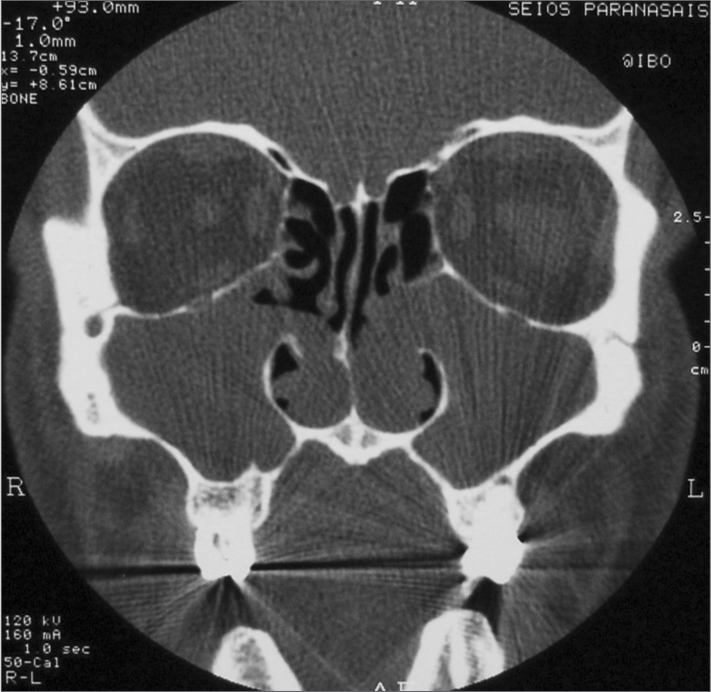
CT of the paranasal sinuses - coronal section showing tumors in both maxillary sinuses, which extend to the nasal cavity through the middle meati.
